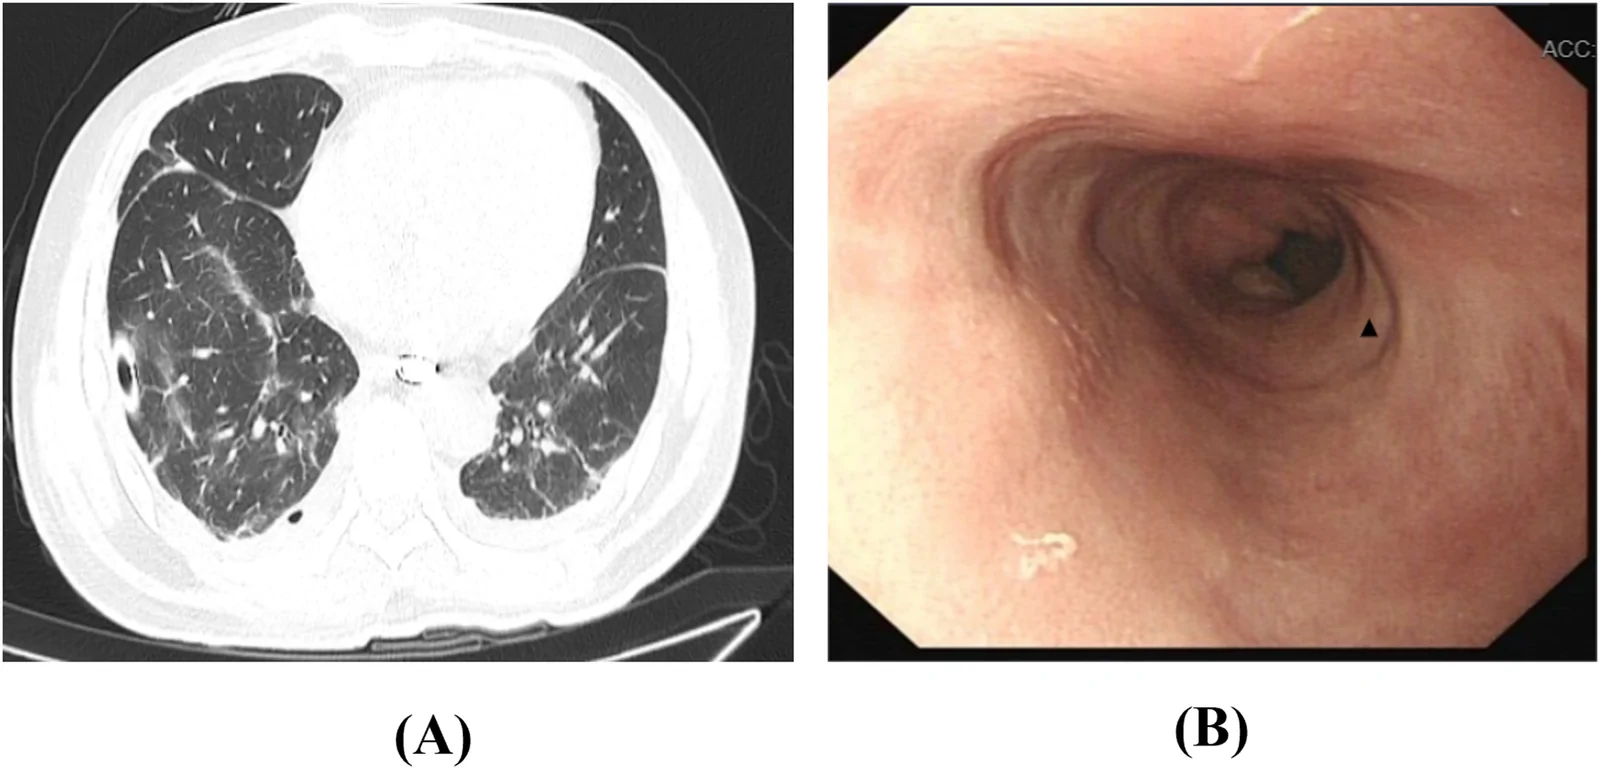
Follow-up evaluation after multidisciplinary treatment. (A) Follow-up chest CT demonstrating marked resolution of pleural contamination and satisfactory lung re-expansion. (B) Endoscopic image confirming complete healing of the esophageal perforation.
